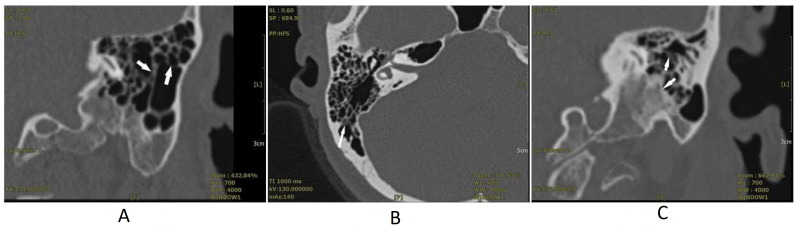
High-resolution computed tomography (HRCT) results. The white arrow indicates the following: mucosa thickening and bony sequestration of air cells in the coronal view of Mr. H’s HRCT examination (A), mild opacification of the air cells along with the cortical bone and bony sequestration in the axial view of Ms. R’s HRCT examination (B), and soft tissue density in the middle ear cavity, mild opacification and bony sequestration of the mastoid air cells along with the cortical bone in the coronal view of Mrs. C’s HRCT examination (C).
